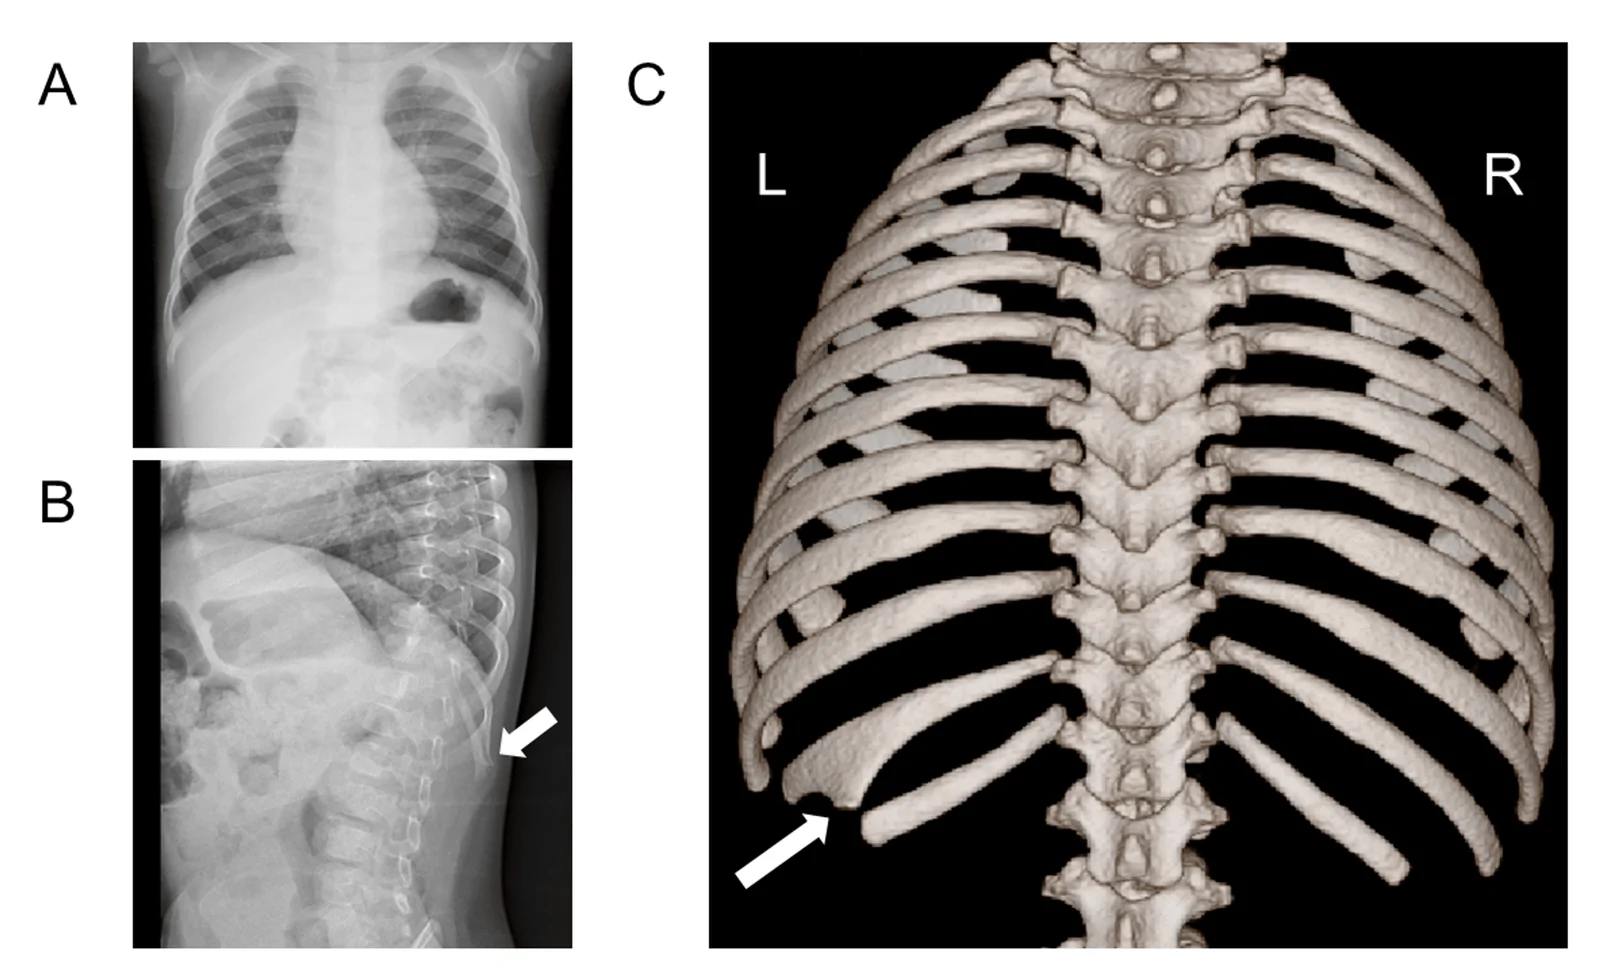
Imaging studies leading to the diagnosis of a bifid left 11th rib. (A) Anteroposterior chest radiograph showing no obvious abnormality of the left 11th rib. (B) Oblique rib radiograph demonstrating a triangular bony projection (arrow) arising from the left 11th rib. (C) Three-dimensional computed tomography demonstrating bifurcation of the left 11th rib (arrow). The posteriorly protruding branch corresponded to the palpable dorsal mass. R and L indicate the right and left sides, respectively.
